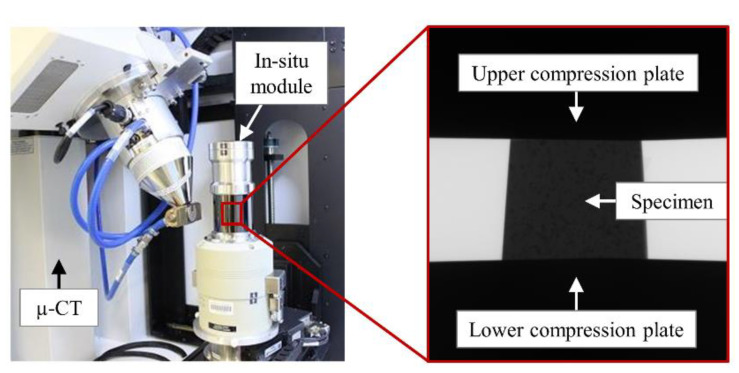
In situ CT testing setup.
